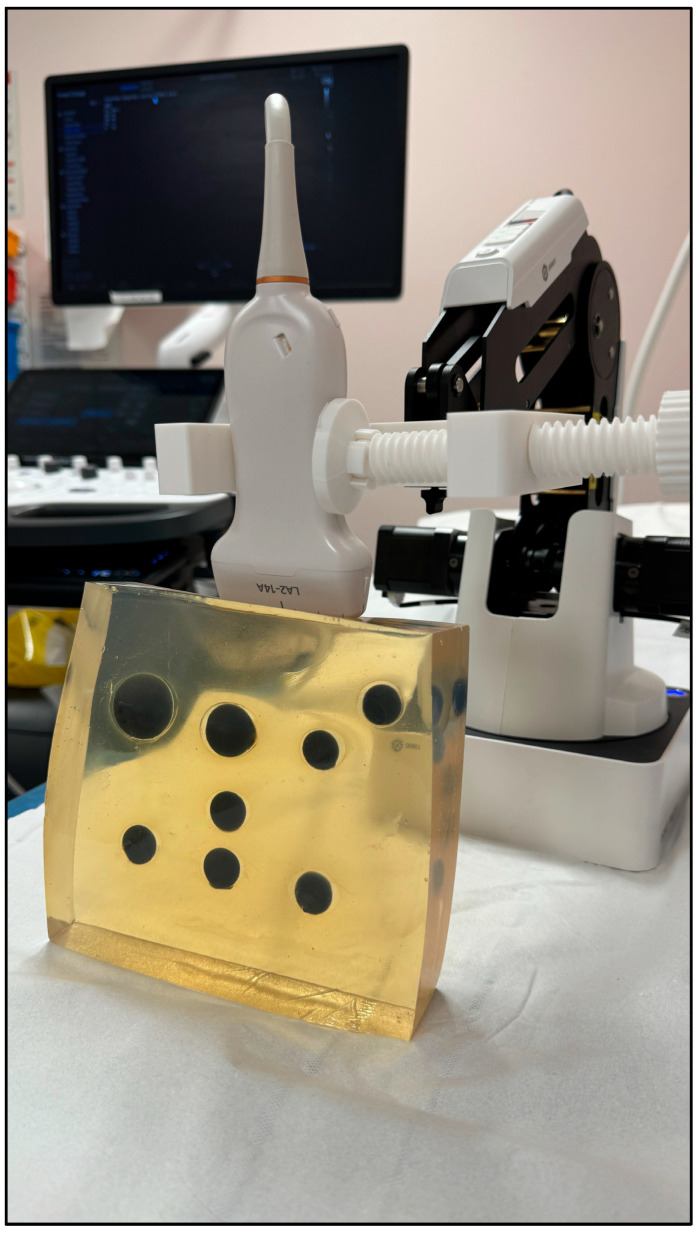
During shear wave elastography scanning on machine A, a robotic arm is used to ensure consistent positioning, orientation, and contact pressure of the ultrasound probe. The robotic arm controls both vertical and lateral movements of the probe, eliminating operator variability.
